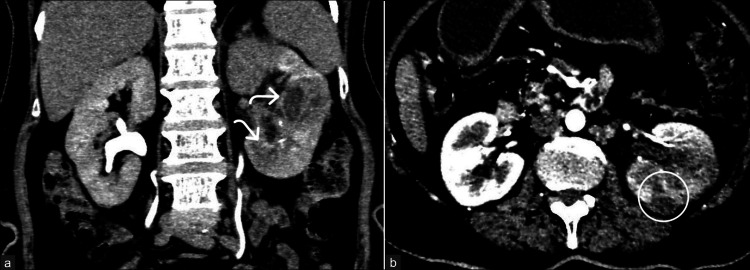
Acute pyelonephritis complicated by multifocal renal abscesses. A 55-year-old woman complaining of left loin pain and dysuria was diagnosed with acute pyelonephritis of the left kidney with multifocal renal abscesses. (a) Coronal reformatted image in the delayed phase of CT urogram shows relatively enlarged left kidney with edematous cortex and multifocal heterogeneous peripherally enhancing cystic areas (curved arrows), suggestive of abscesses. (b) Post-processing brightness-adjusted axial image in the delayed phase shows the rupture of one of the cystic lesions (circle) in the lower pole. These images are from the hospital and have not been published elsewhere. CT: computed tomography
